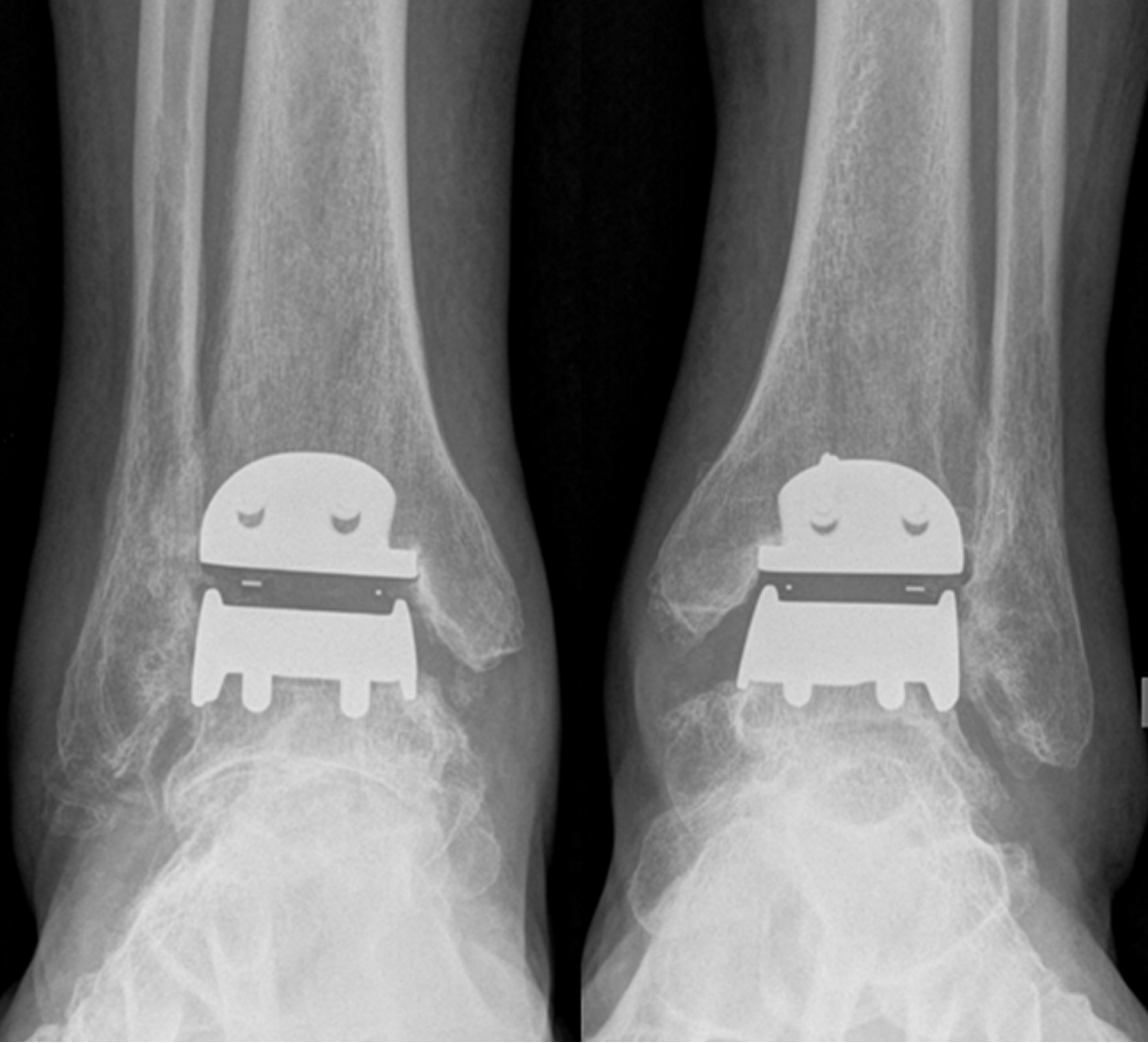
Anterior radiographs of both ankles 25 months after TAA. The picture shows bilateral TAAs with corrected varus alignment.
